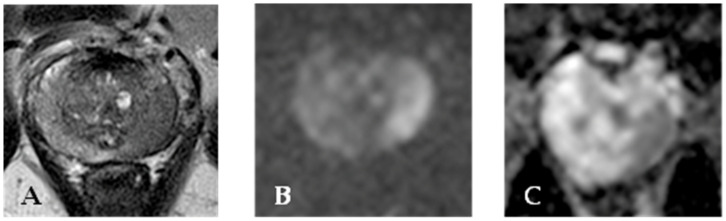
MR of a peripheral left lobe PC. T2 weighted sequence (A) shows an area of hyperintensity in the left lobe. This finding is confirmed on apparent diffusion coefficient sequences (B). DWI sequence (C) reveals hyperintensity in the lesion area.
